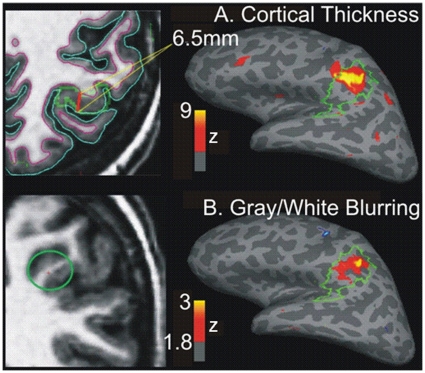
Cortical dysplasia marked by cortical thickening and gray-white boundary blurring in Patient 3. A. Significantly increased thickening of the cortical ribbon (in red/yellow) detected by the automated quantitative approach falls within the expert-delineated area of focal cortical dysplasia (green tracing on the inflated pial surface). The area with highest z-score shows cortical thickness measures of up to 6.5 mm between white matter and pial surfaces. B. Calculating the T1-signal contrast at .5 mm above vs below the gray/white interface shows an area of significant blurring within the lesion. The green circle on the left shows the area of maximum blurring on a coronal volumetric MRI slice.
